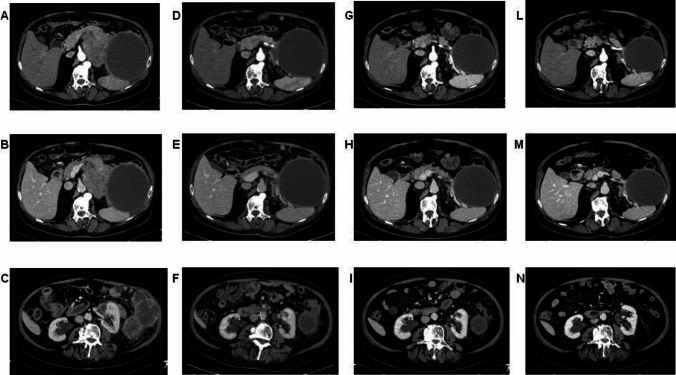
CT scans after two lines of chemotherapy, before the start of pembrolizumab (A, B, C). CT scans after 3 months (D, E, F), after 6 months (G, H, I) and after 10 months (L, M, N) of pembrolizumab
